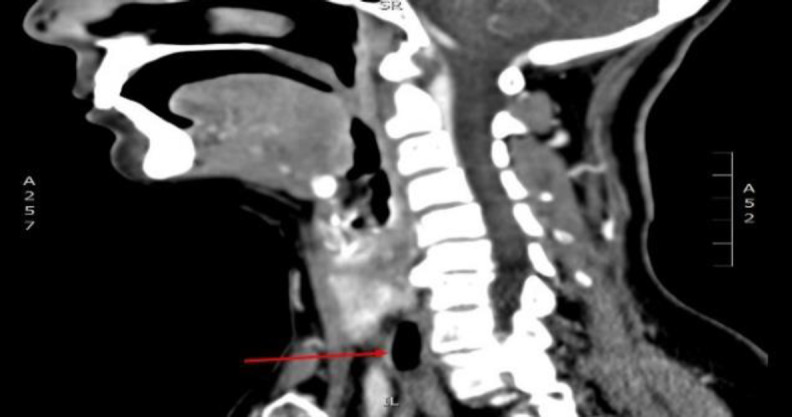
Neck CT (sagittal scan) showing DISH at C5-C6 level and Zenker's diverticulum at C7 level (red arrow)
